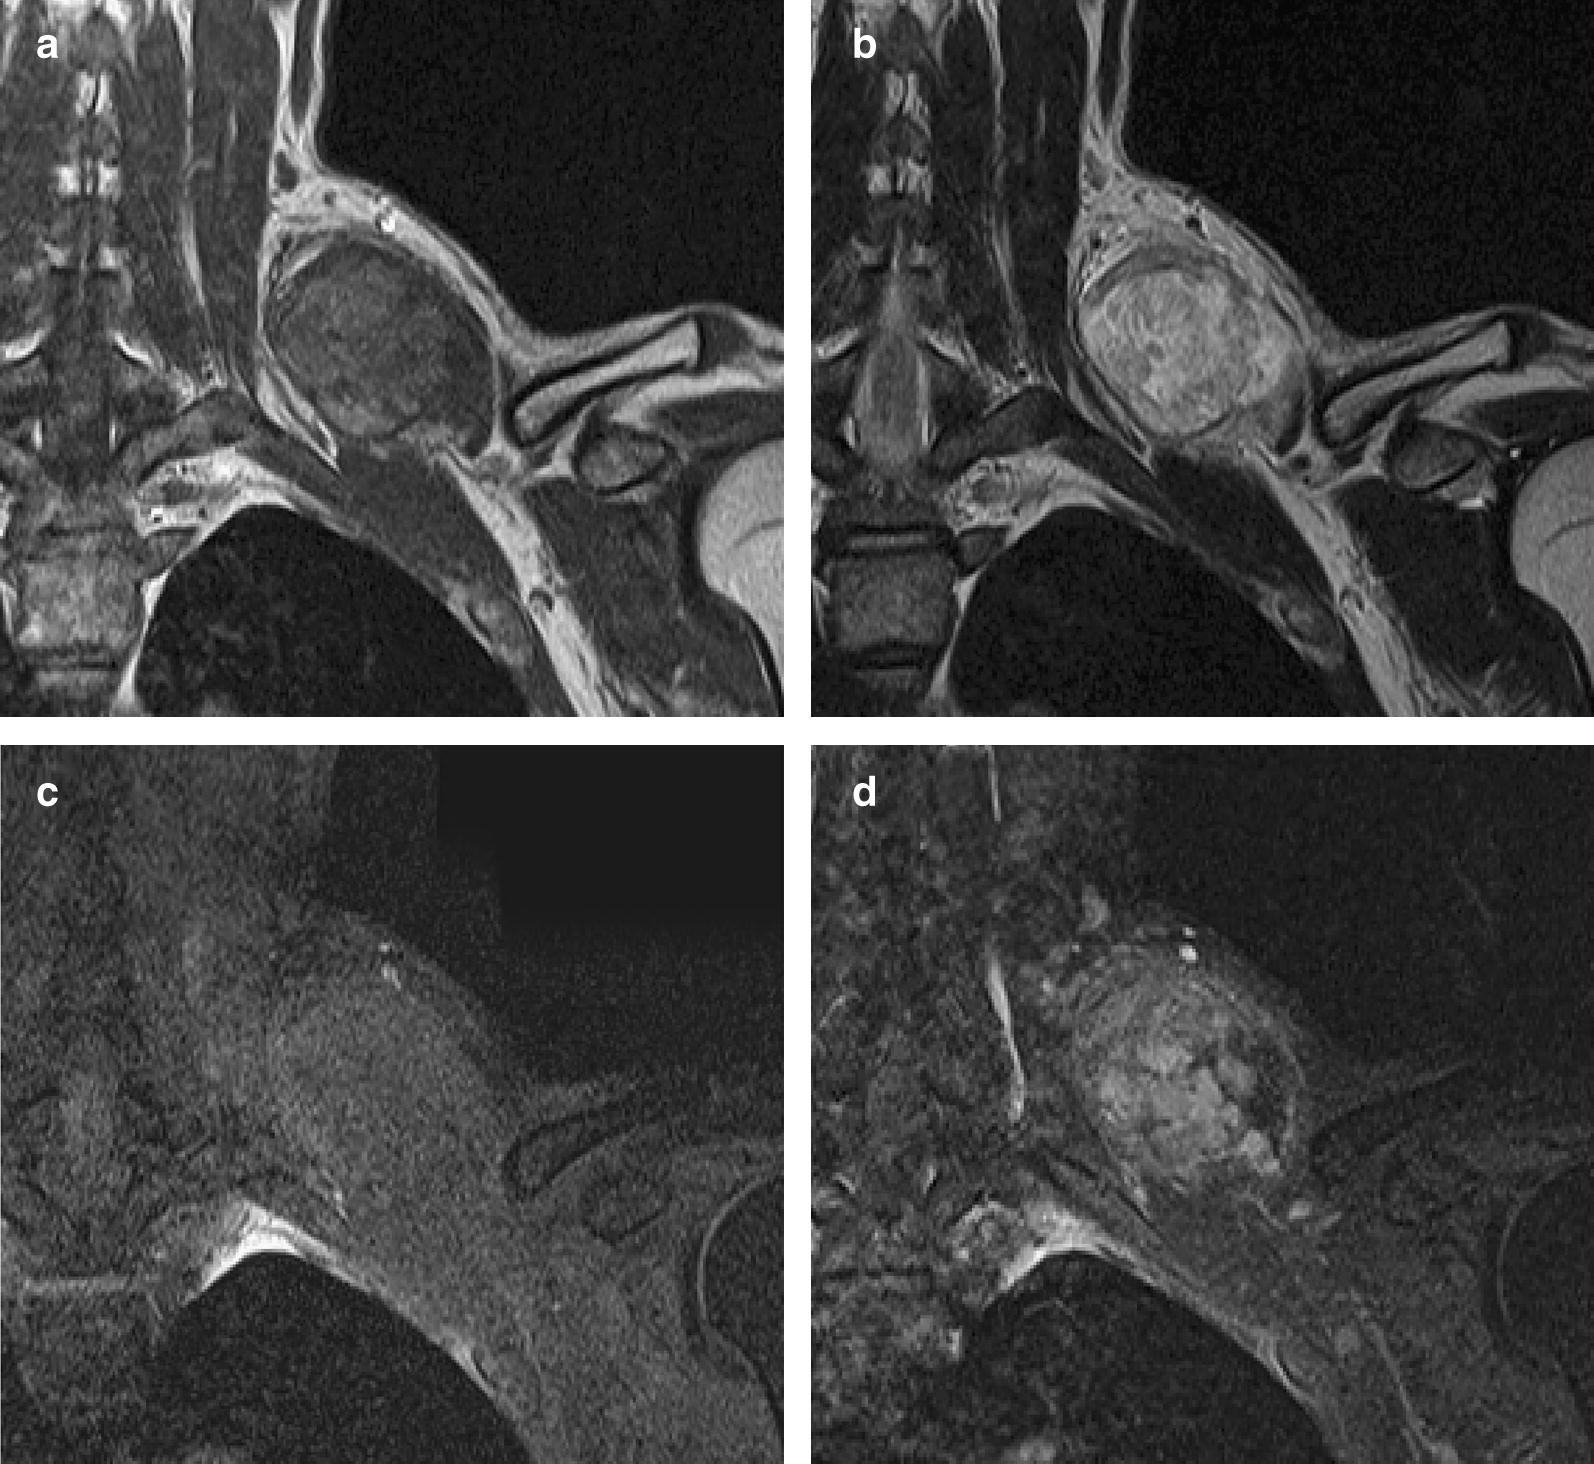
MR imaging shows a well-defined and lobulated mass on the left neck. a On T1-weighted images, the mass has heterogeneity with high-signal intensity than muscle. b On T2-weighted images, the septum has low-signal intensity. c On T2-weighted fat-suppressed images, the signal of the mass is suppressed totally. d On T1-weighted images following gadolinium administration, the mass is enhanced
